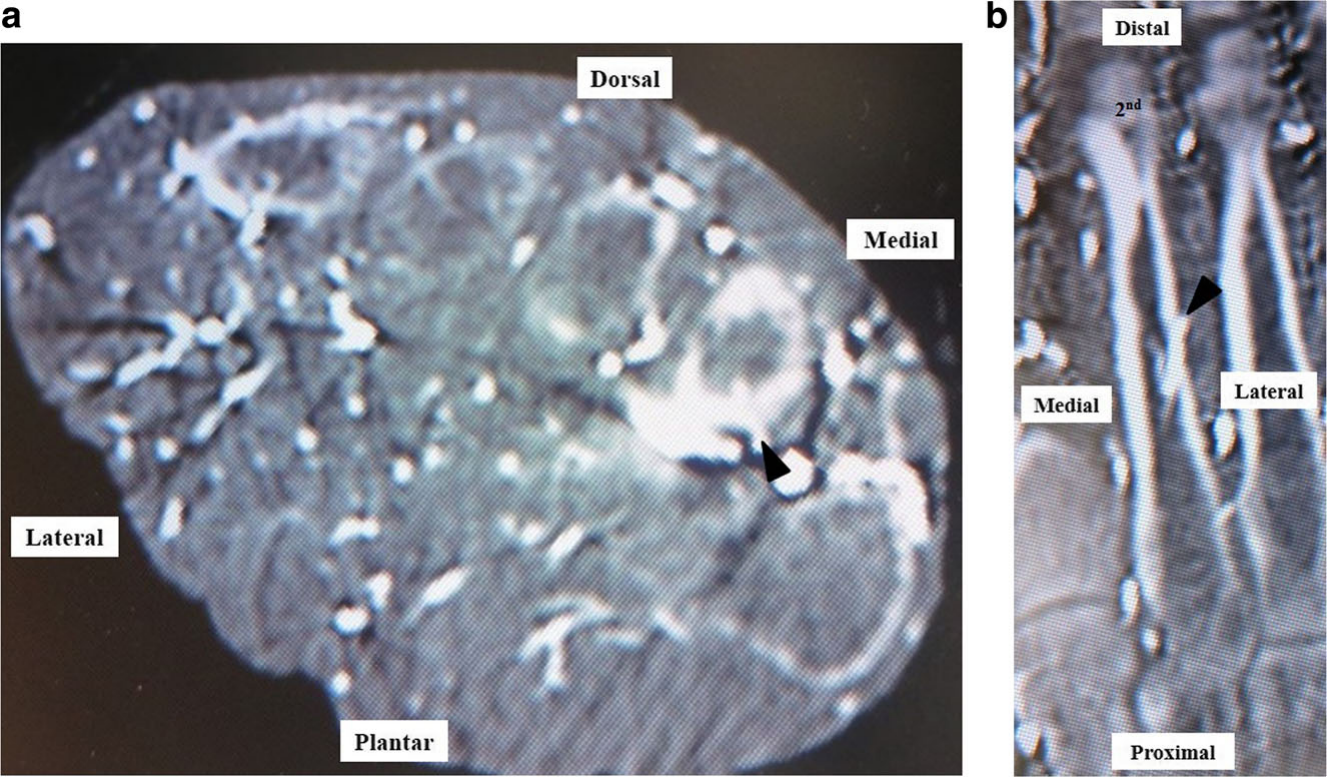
Enhanced CT images showing the nutrient artery entering the lesser metatarsal medially (a) In the axial plane and, (b) in the coronal plane. The arrow head indicates the nutrient artery
